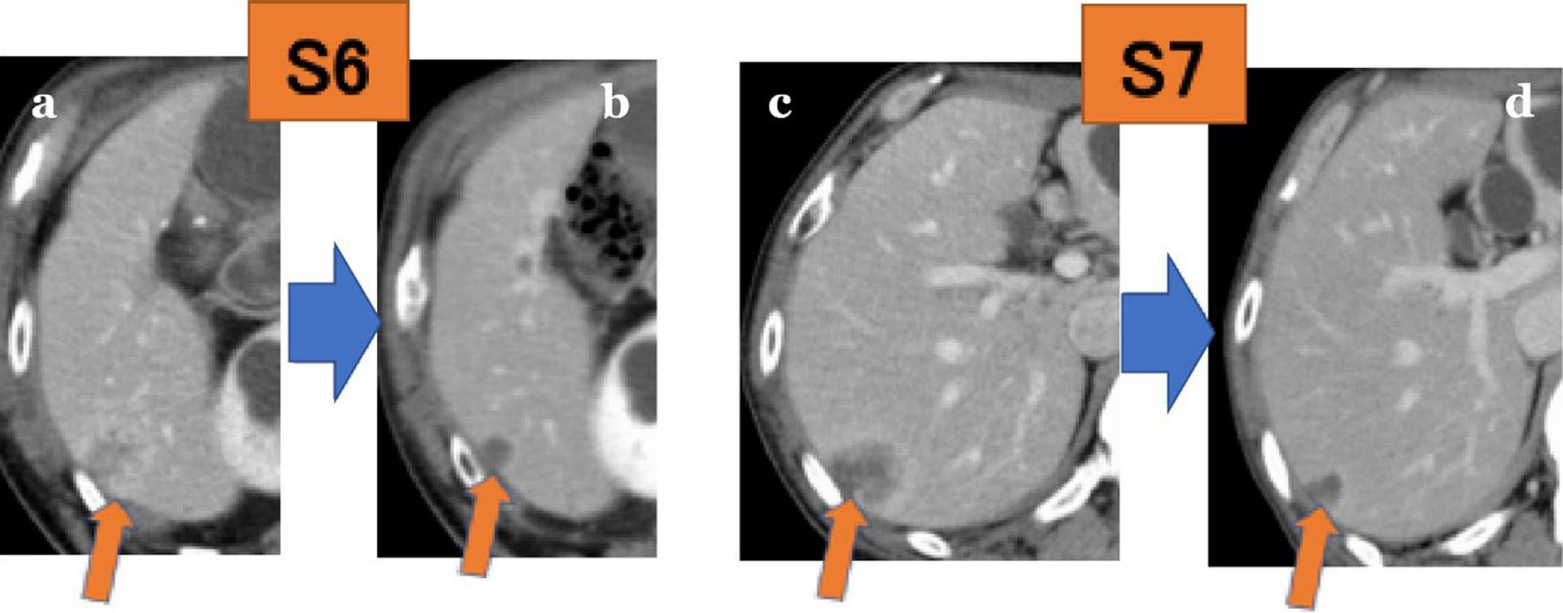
Computed tomography images before and after lenvatinib plus pembrolizumab treatment. (orange arrow) a Metastatic tumor in segment 6 before treatment. b Metastatic tumor in segment 6 after treatment. c Metastatic tumor in segment 7 before treatment. d Metastatic tumor in segment 7 after treatment
